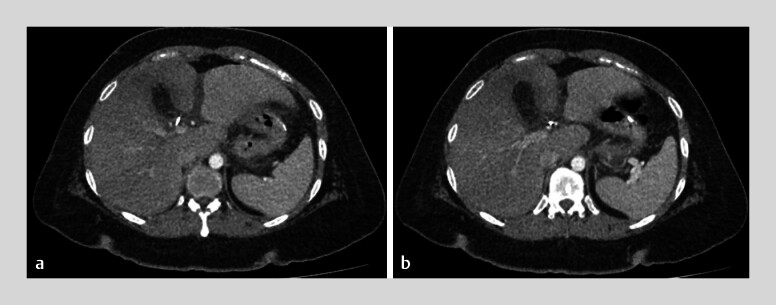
Abdominopelvic computed tomography scan 10 days after surgery showing on axial sections the cholecystectomy clips, with fat infiltration in the cholecystectomy site, but no bile duct dilatation.
